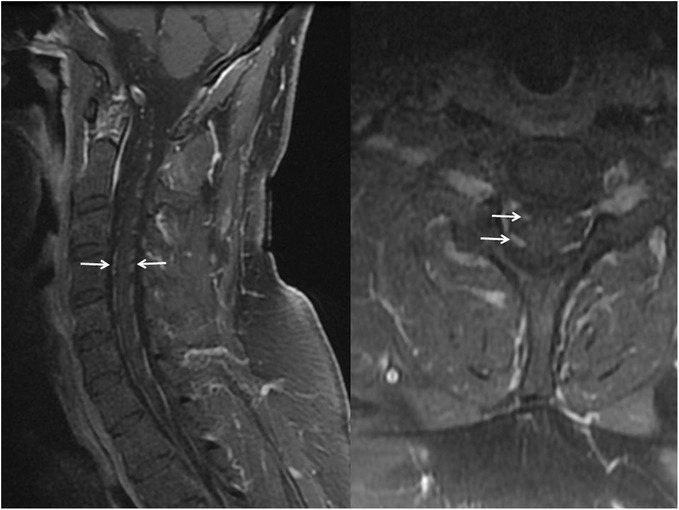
Follow up post contrast cervical spine MRI. Parasagittal and axial T1w fat saturated post contrast images demonstrating smooth avid enhancement of all anterior and posterior cervical nerve roots (arrows) but no involvement of the cord surface. Both factors strongly mitigate against leptomeningeal metastatic disease
